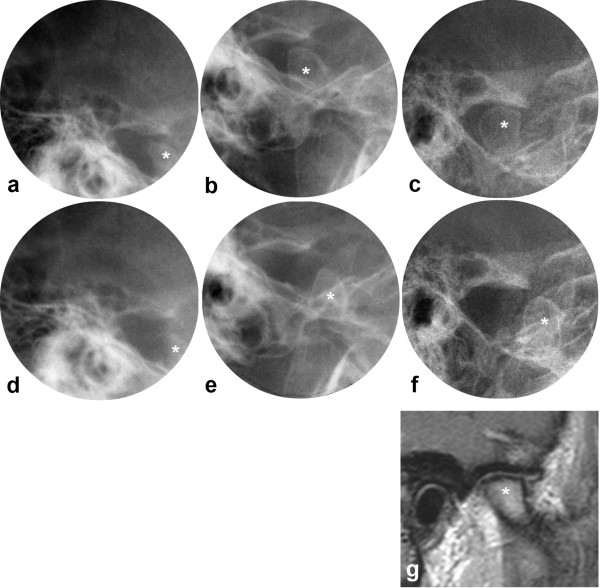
JIA patient with greatly improving disease course in the TMJs, right joint. Transcranial radiographs at closed (a,b,c) and maximally opened mouth (d,e,f), including MRI (g) at closed mouth. Right joint shown from 5 to 33 yrs with extensive bone abnormality and severely impaired condyle translation at baseline (a,d), improved condyle morphology and translation at 2 yrs follow-up (b,e), and further improvement of morphology and translation at final follow-up (c,f,g). Note the normalization of the condyle position in the fossa at closed mouth between the first and last examinations. * = mandibular condyle. Mandibular growth rotation shown in Fig. 2c.
